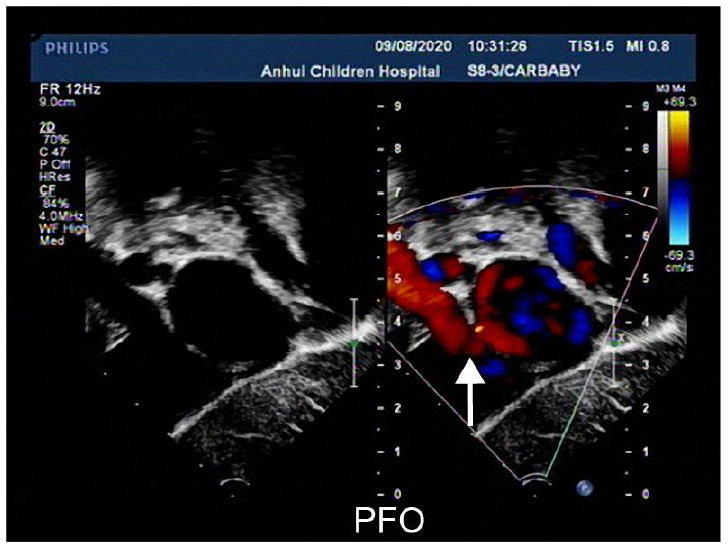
M-mode echocardiography: LVDD 1.8, LVDS 1.1 EF74%, FS40%, Two-dimensional echocardiography: ASD(secundum type,0.36cm), PDA is small, two dimensional echocardiography is not clear. Doppler echocardiography: ASD L → R(+), PDA L → R a small amount of shunt, MR(-), TR(+)mild regurgitation, PI(+)mild regurgitation, AI(-)	M-mode echocardiography: LVDD 1.7, LVDS 0.9, EF81%, FS47%; ASD(secundum type, 0.38cm), the ventricular septum of the heart was thickened, and the thicker middle part was 1.5cm,PDA is small, two dimensional Doppler echocardiography: LVOT2.7m/s, MPA2.1m/s, LPA2.6m/s PDA L → R a small amount of shunt,ASD L → R, MR(-),TR(+)mild regurgitation, PI(+)mild regurgitation,AI(-)	M-mode echocardiography: LVDD 2.2, LVDS 1.2 EF78%,FS44%, PFO Doppler echocardiography: AAO 1.0m/s,MPA3.0m/s PFO L → R(+), MR(-), TR(+)mild regurgitation, PI(+)mild regurgitation AI(-)
